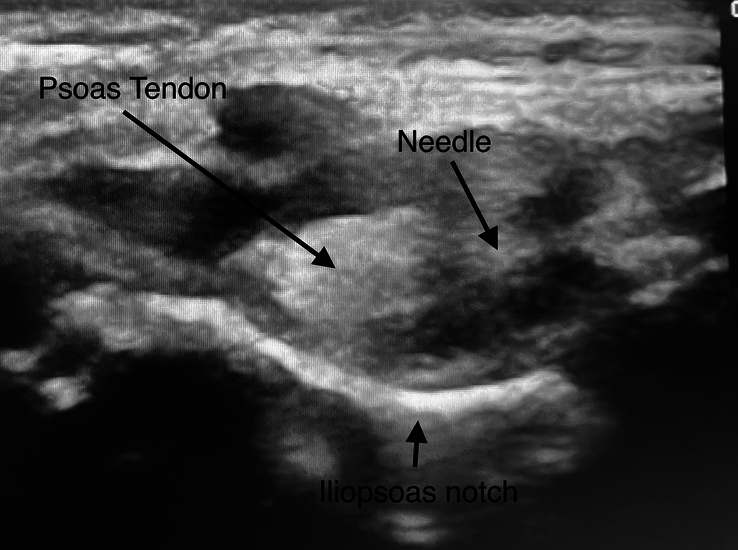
Ultrasound-guided PENG block. PENG indicates pericapsular nerve group.
